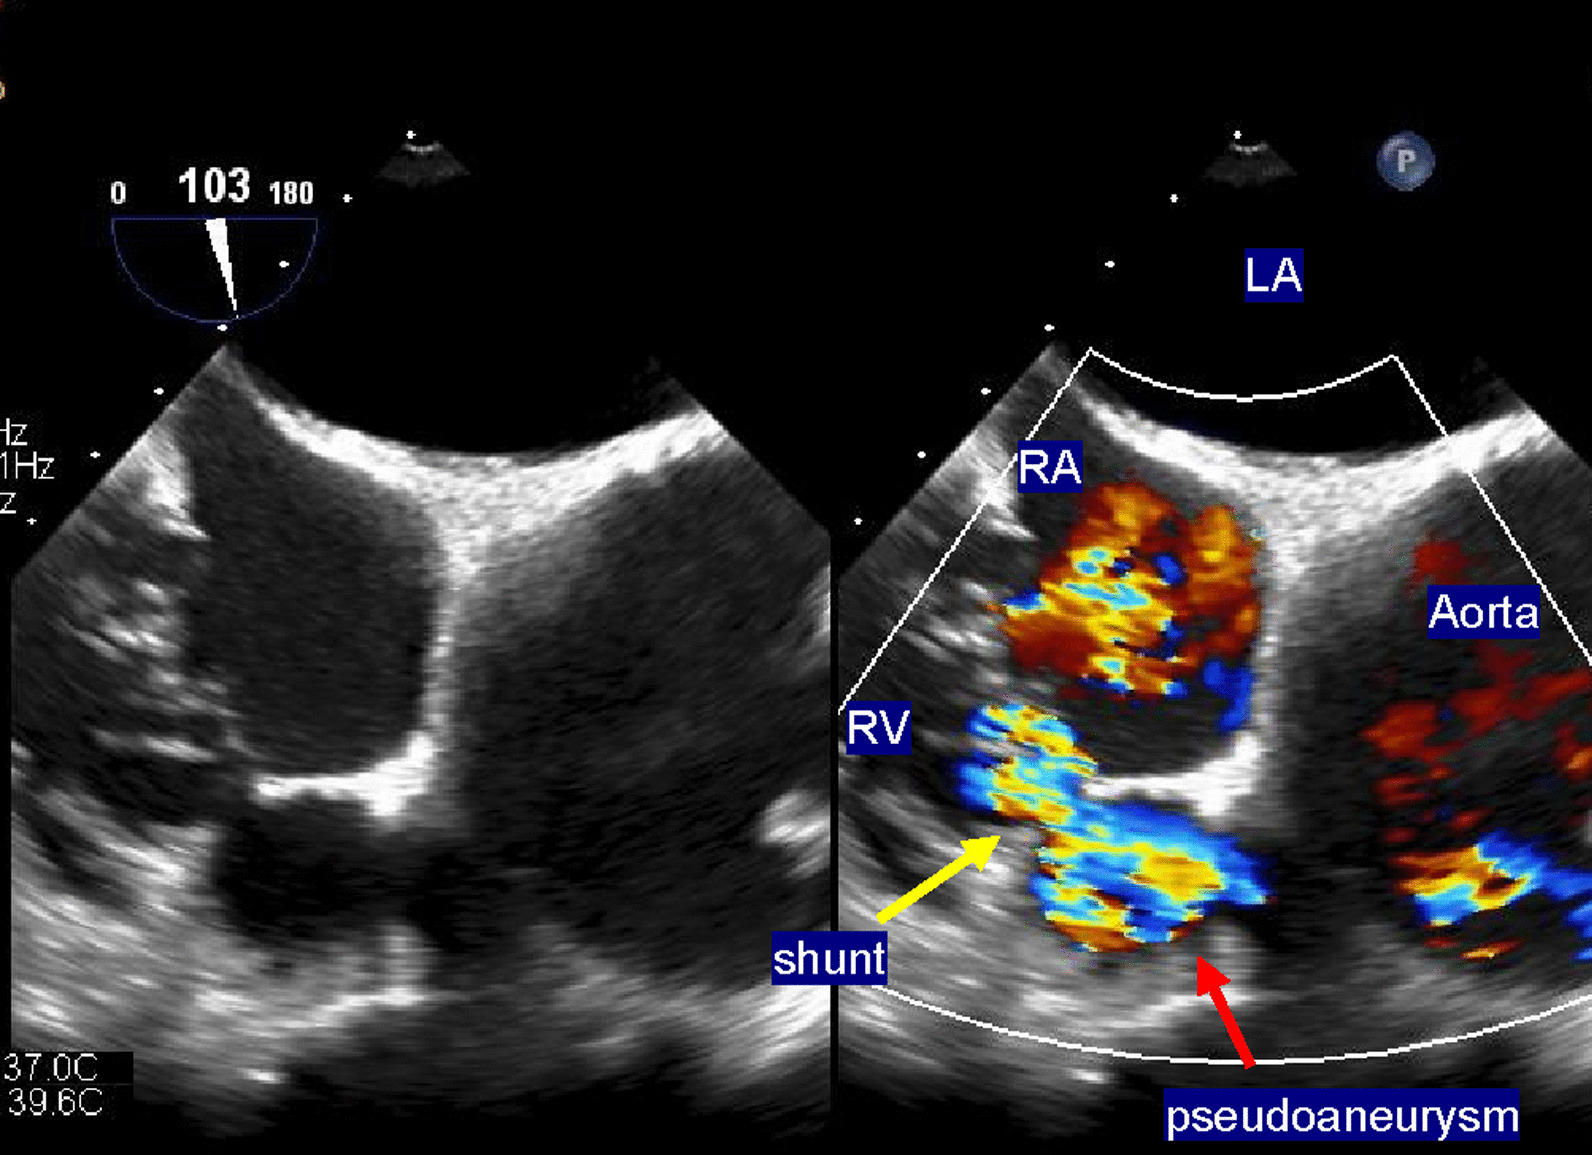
Transesophageal cardiac ultrasonography showing the pseudoaneurysm (red arrow). The shunt (yellow arrow) is indicated. RA, right atrium; RV, right ventricle; LA, left atrium
